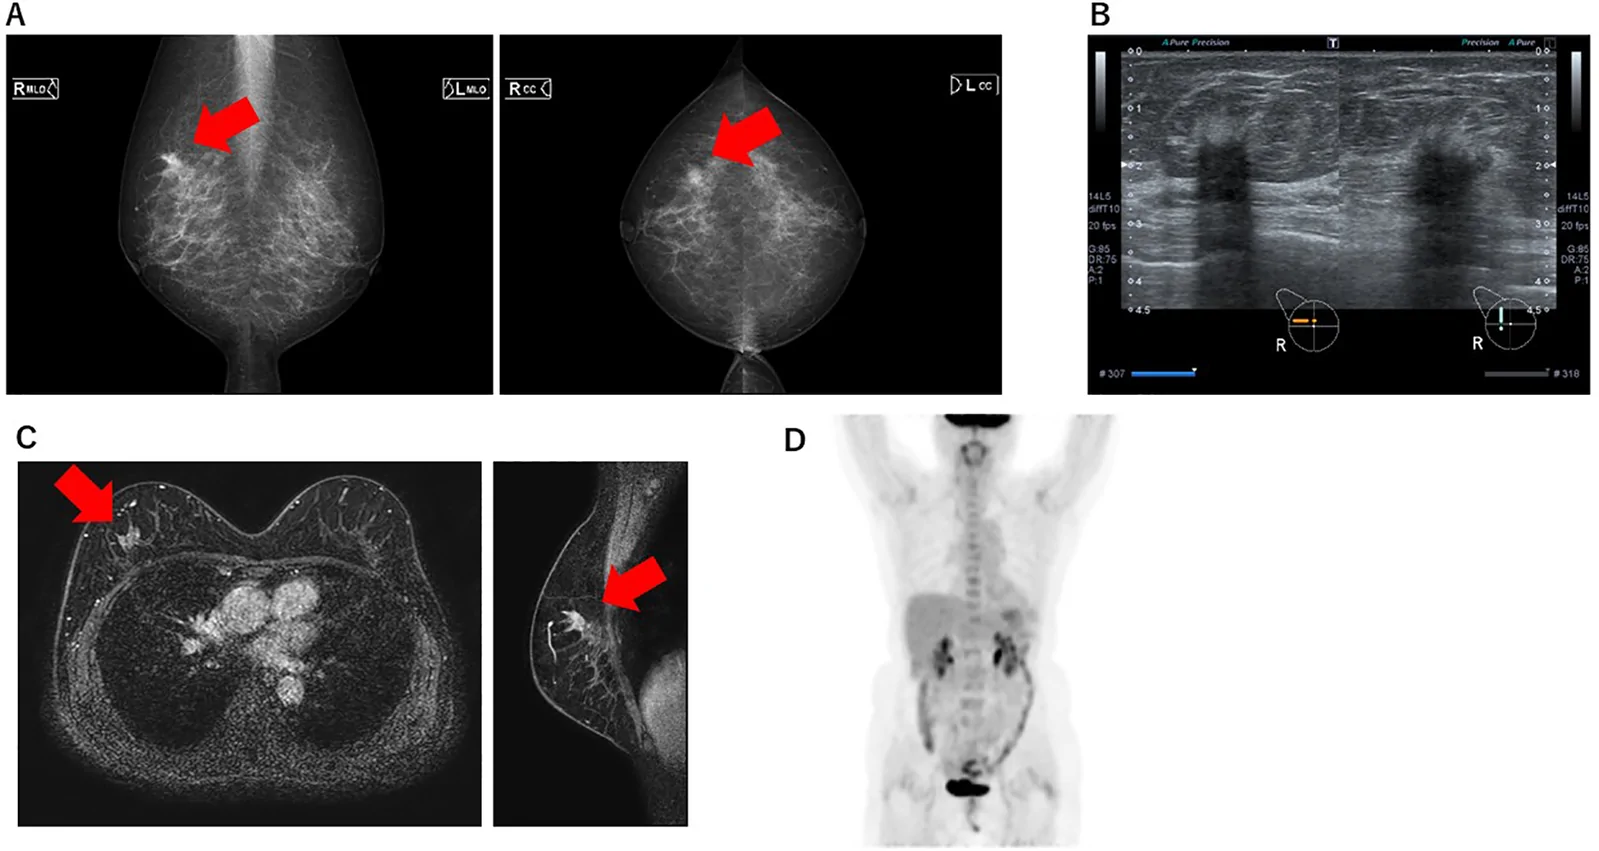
At diagnosis of the right breast cancer. The red arrow indicates the tumor. (A) On mammography, an irregular mass with spiculated margins causing architectural distortion was identified in the upper outer quadrant of the right breast. (B) Breast ultrasonography demonstrated a hypoechoic mass at the corresponding site with posterior acoustic shadowing and features suggestive of infiltrative growth into the surrounding fatty tissue. (C) Magnetic resonance image also revealed a mass with surrounding tissue invasion; however, there was no marked early enhancement on dynamic imaging, and a gradual enhancement pattern was observed. (D) On positron emission tomography, no definite distant metastasis were identified. The right breast tumor showed an SUVmax of 1.2 on FDG-PET/CT.
